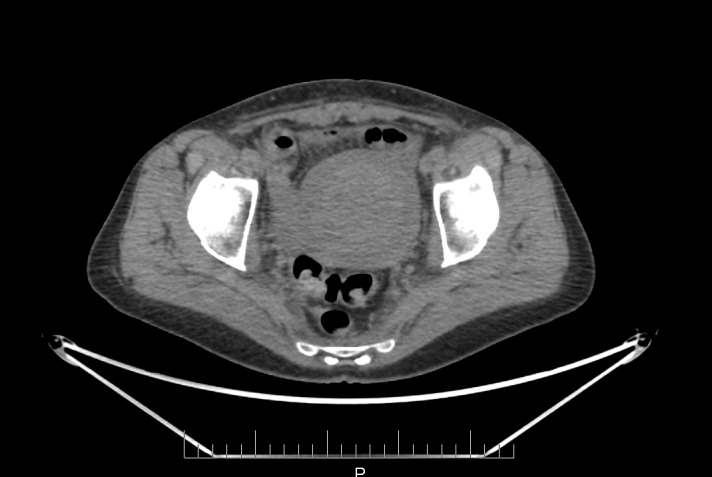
CT scan transverse image from the PET-CT showing normal thickness of the sigmoid, 3 months post-radiation
